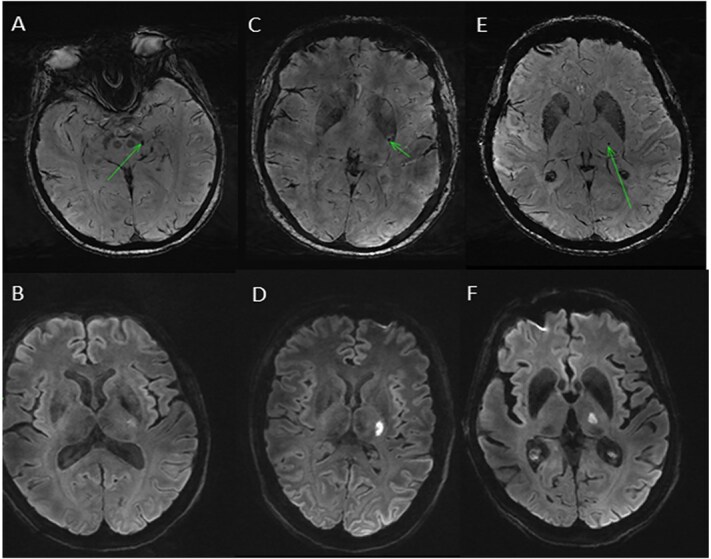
(A–F) Susceptibility-weighted imaging (SWI) demonstrating likely susceptibility vessel sign (SVS) in three different patients and associated diffusion-weighted imaging (DWI) hyperintensity representing acute infarct.
